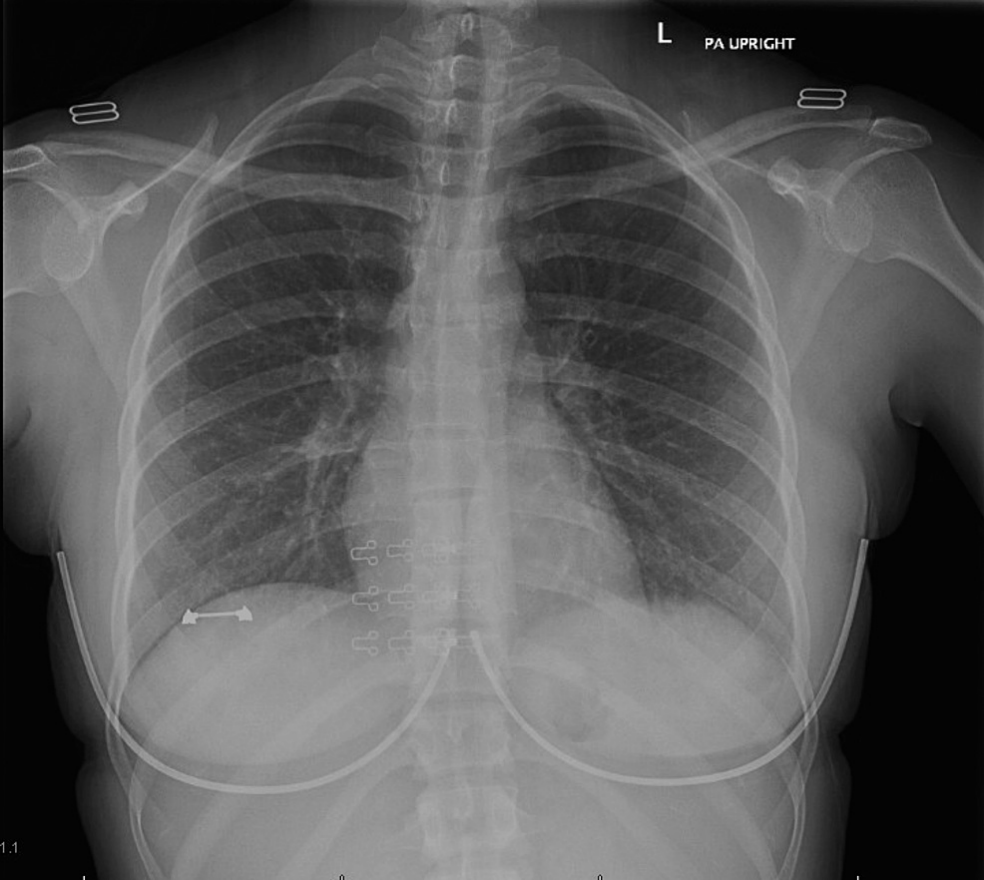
CXR demonstrating clear lungs and pleural spaces. No radiographic evidence of acute pulmonary disease.
